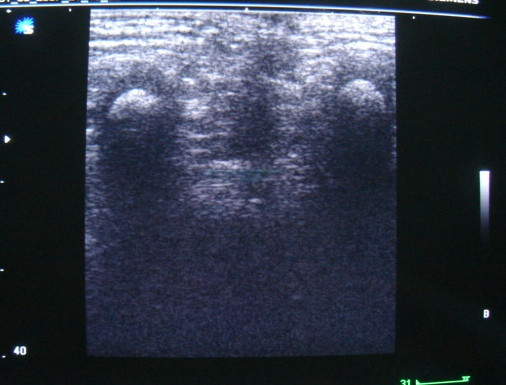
Preoperative localization of the center of muscle complex. A perineal sonogram showing muscle complex on two sides with variable amount of levator fat.
